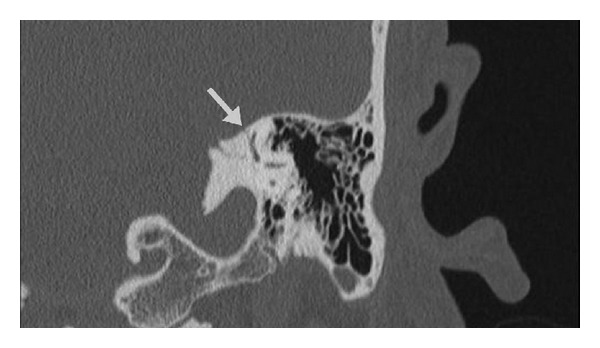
Computed tomography (CT) imaging of the patient in Case 1. Coronal section demonstrates a left temporal bone fracture (arrow) traversing the bony superior semicircular canal.
